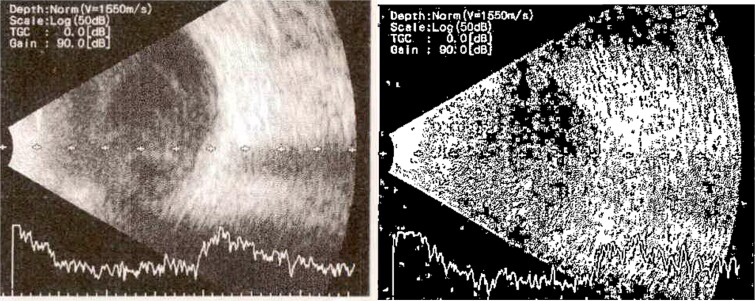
B-scan ultrasound showing moderate vitreous echoes and vitreous abscess in patient 2.
